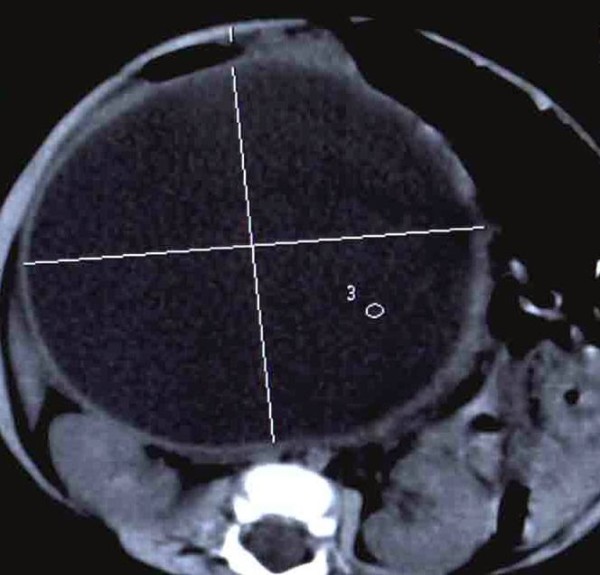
This CT scan shows irregular dilatation of common bile.
